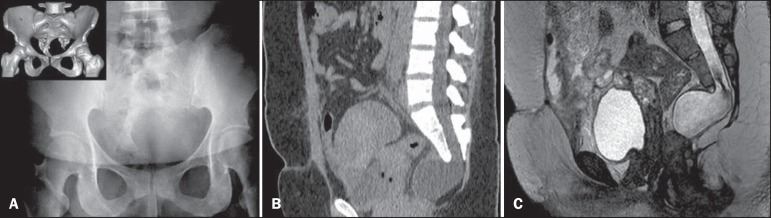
A: Conventional radiography showing unilateral sacral curvature
